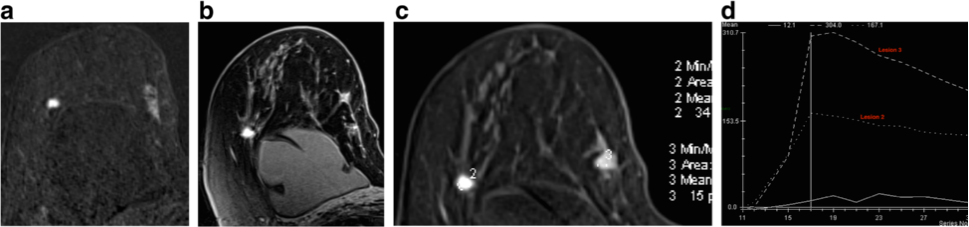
Patient with known grade 1 classical invasive lobular carcinoma in the upper inner quadrant of the right breast. MRI was indicated as the patient had breast implants and the breasts were difficult to assess with conventional imaging. Unexpected finding of a second carcinoma in the upper outer quadrant. a Post contrast subtracted image, (b) high resolution delayed post contrast image, (c) regions of interest and (d) time-intensity curves. Note different morphology and kinetics of the two lesions; lesion 3 in the lateral breast was a hormone receptor positive grade 3 invasive ductal carcinoma
